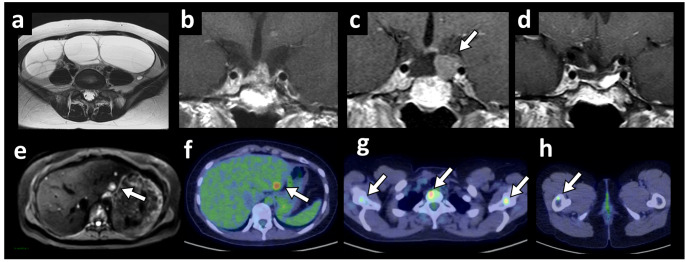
Radiographic findings of case 1. a Abdominal MRI T2-weighted image (T2WI) showing bilateral ovarian enlargement and polycystic follicles. b Head MRI T1-weighted image (T1WI) with gadolinium (T1-Gd) enhancement showing no remarkable recurrence of the pituitary tumor at age 20. c Head MRI T1-Gd showing tumor regrowth in the left cavernous sinus at age 28. d Head MRI T1-Gd showing no obvious tumor recurrence in the pituitary at age 35. e Abdominal MRI diffusion-weighted image showing multiple masses in the liver. f–h 18 F-fluorodeoxyglucose positron emission tomography (FDG-PET) scan showing metastases to the liver (f), multiple bone metastases in the cervical, thoracic, and lumbar spine, both scapulae (g), and the right femur (h)
